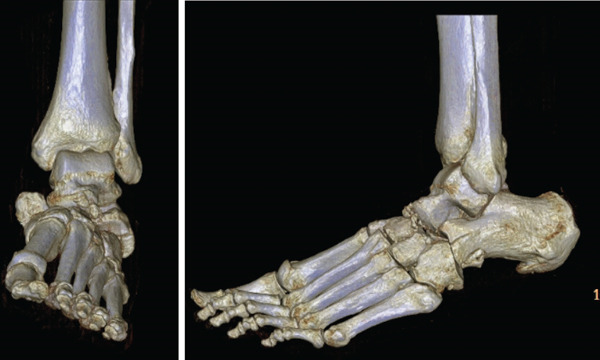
3D reconstruction CT scans of injured foot.
